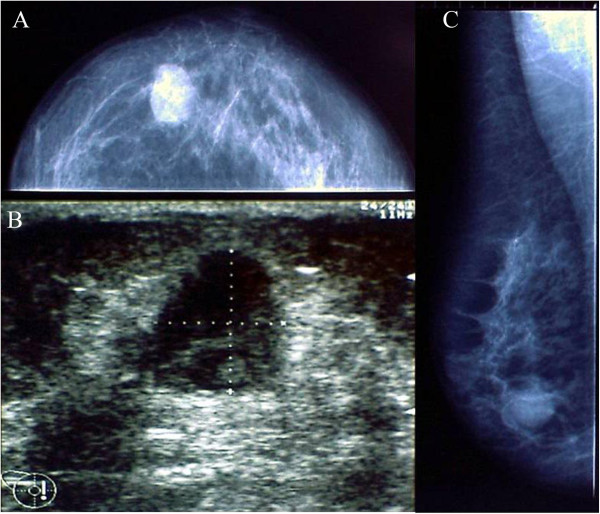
Radiological characteristics of lesion; (A and B) mammography craniocaudal and mediolateral oblique views of the right breast show an ill-defined end opacity in the inner-inferior quadrant of about 20 mm diameter; (C): ultrasonography of the right breast shows a hypoechoic nodule of about 17 mm diameter around the right mammary region near the areola.
